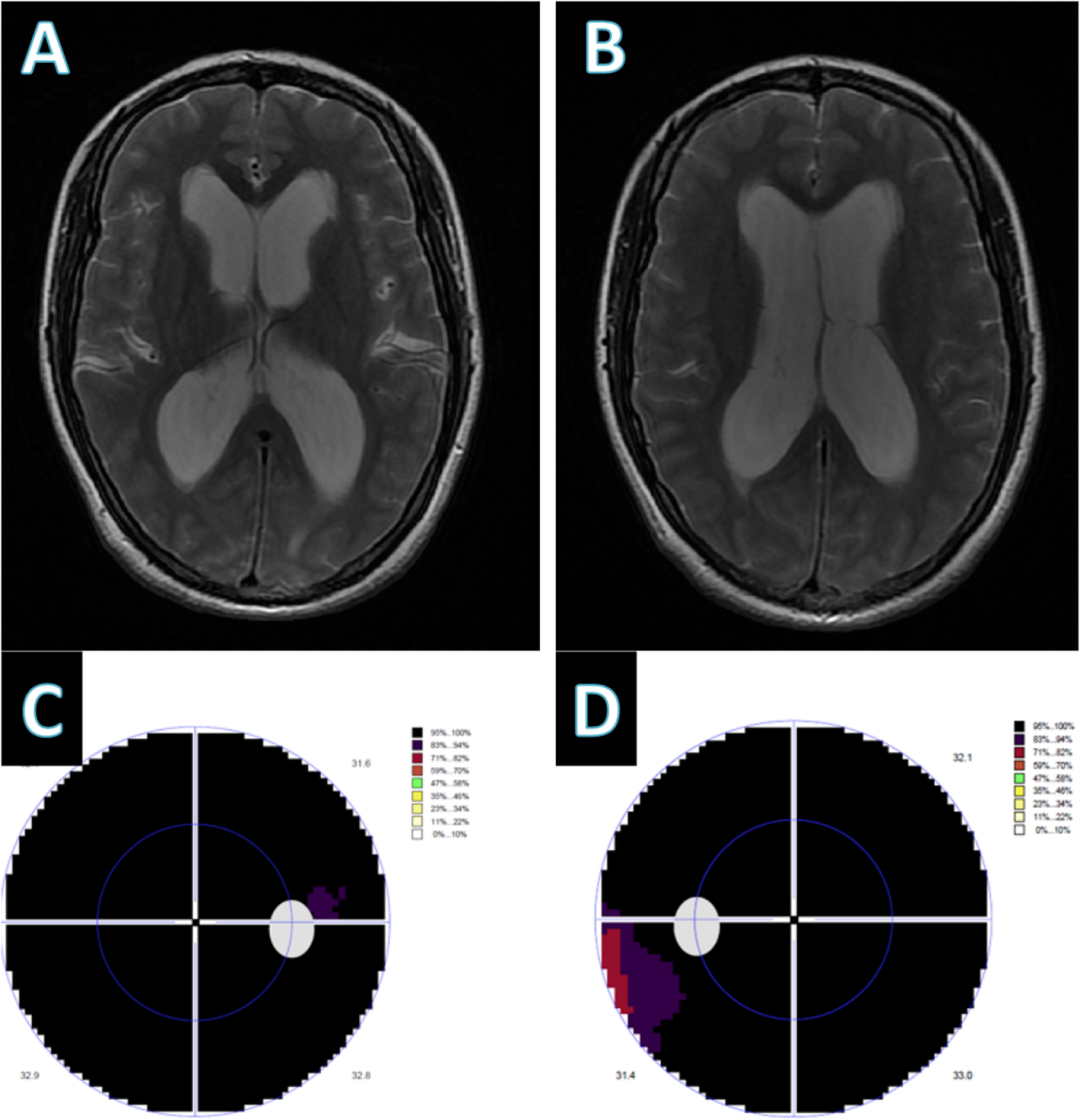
Brain magnetic resonance imaging (MRI) and visual field examination during the second admission 1 year later. a, b Brain MRI showed more pronounced hydrocephalus than before. c, d Ophthalmologic examinations revealed almost complete loss in bilateral visual fields
